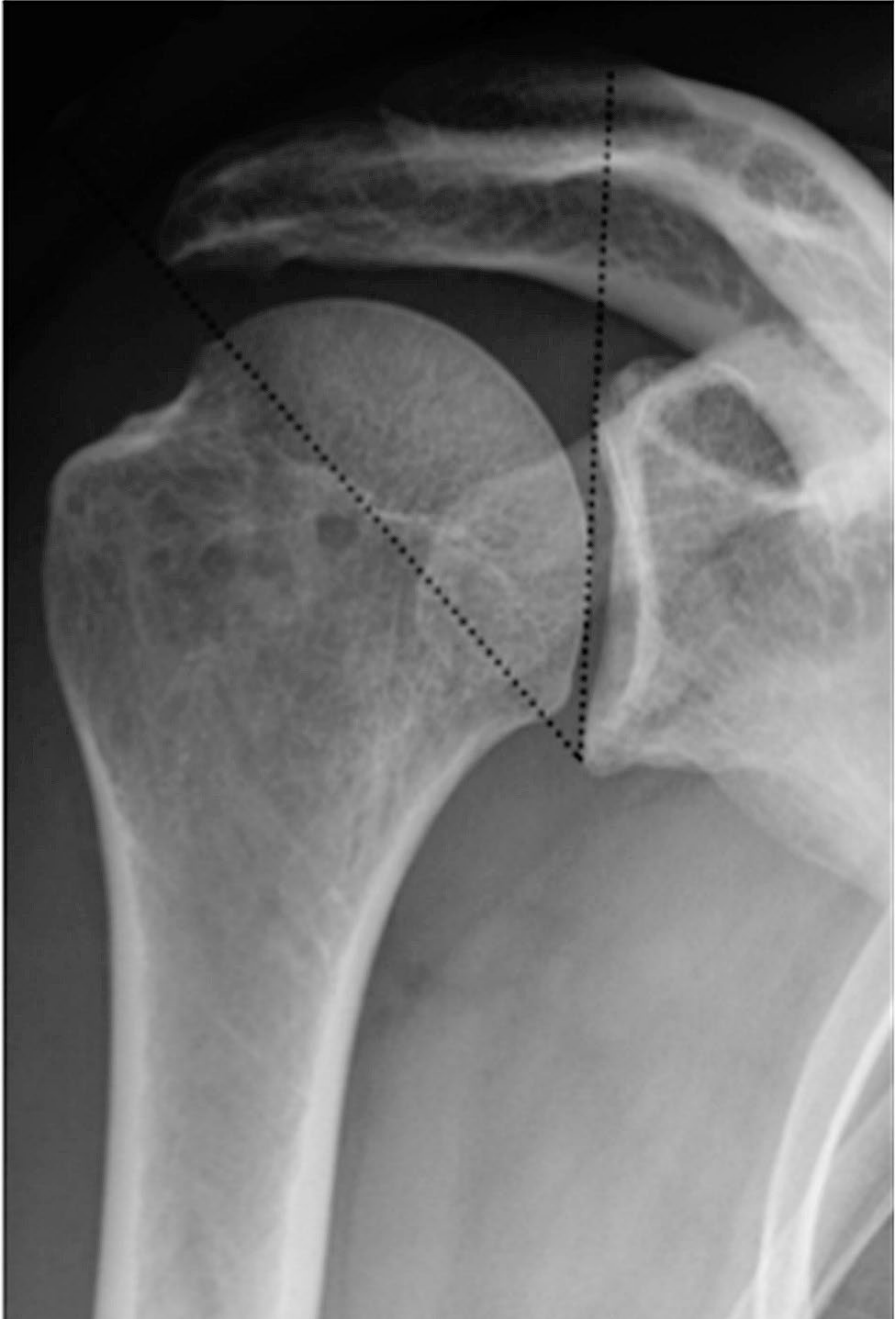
On the anteroposterior X-ray of the shoulder joint, a line is drawn from the upper edge of the pelvis to the lower edge (across the pelvis plane), and a line is drawn from the lower edge of the pelvis to the farthest outer end of the acromion
